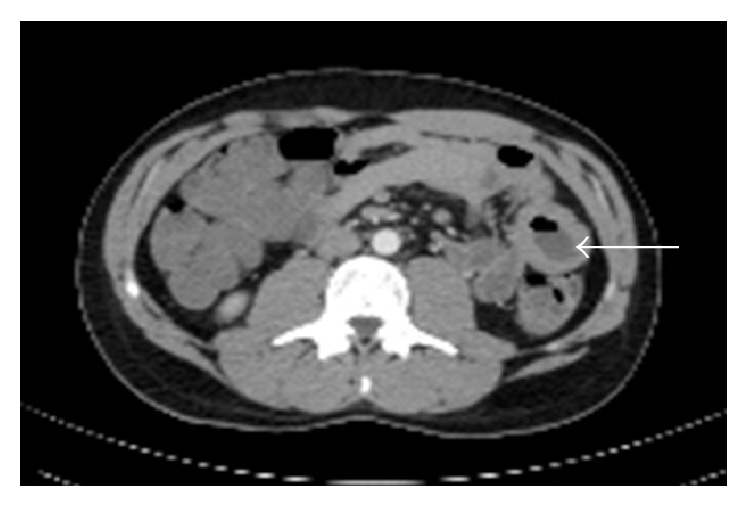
A computed tomography (CT) of abdomen shows lumen thickening with narrowing in distal jejunum (arrow).
